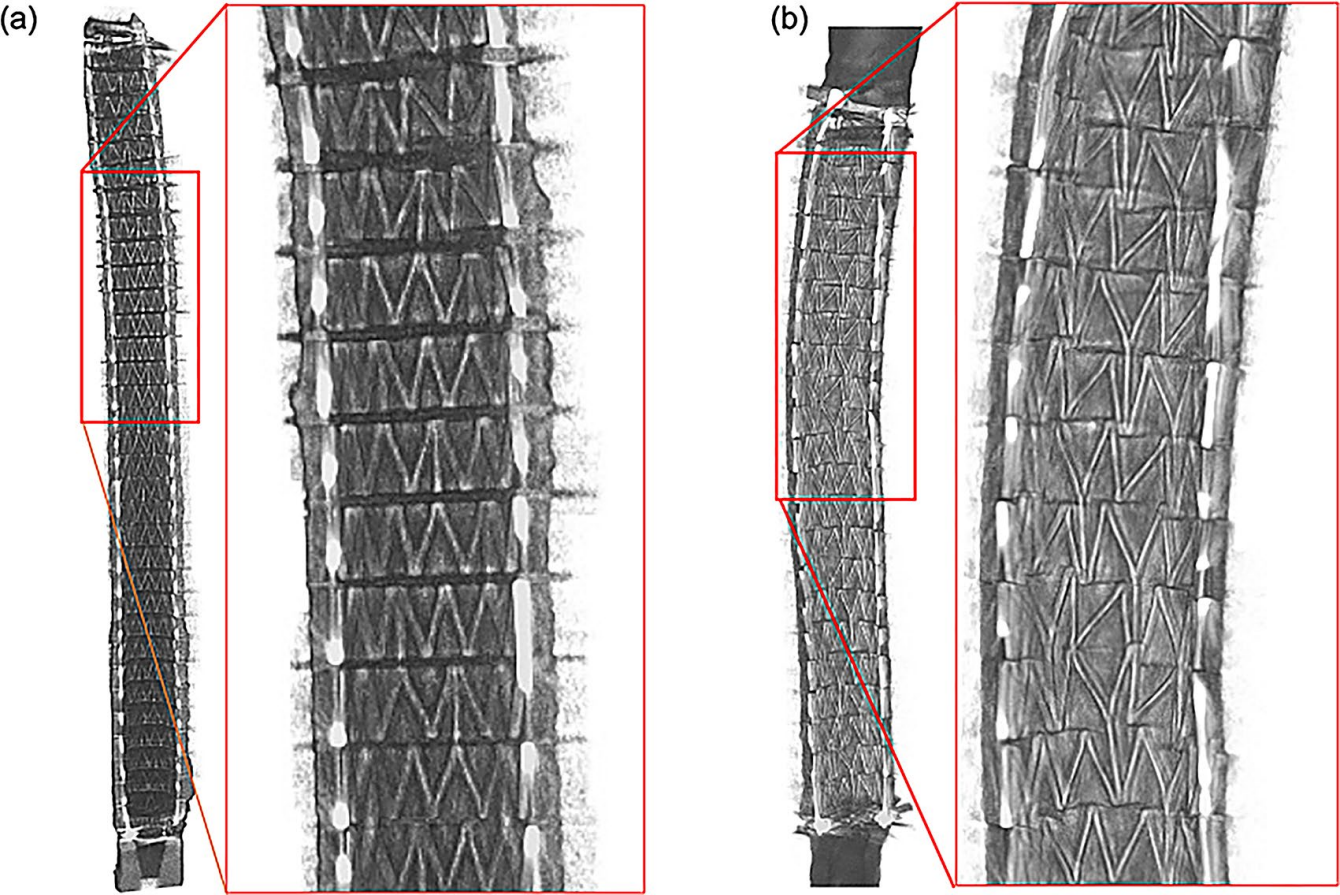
2D Micro CT imaging of the DES under (a) P2 and (b) P3 movements. A more enhanced version also shows the structure and placement of one section (red box) of the stents under these two parameters. P2 (Parameter 2): Pulsatile flow with 16.8° twist, 25° bend, 3.2 mm compression; P3 (Parameter 3): Pulsatile flow with 68° twist, 35° bend, 21 mm compression
